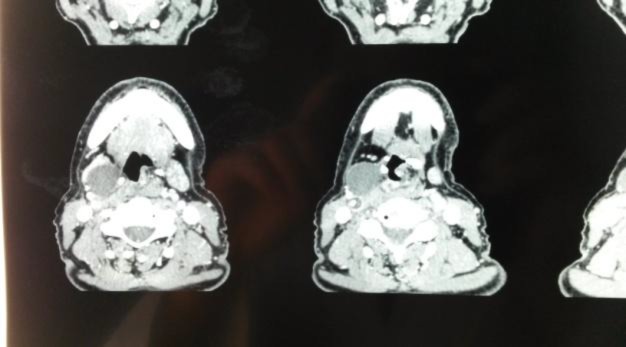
CT-scan: axial view showing hypodense 3.5 cm right formation
